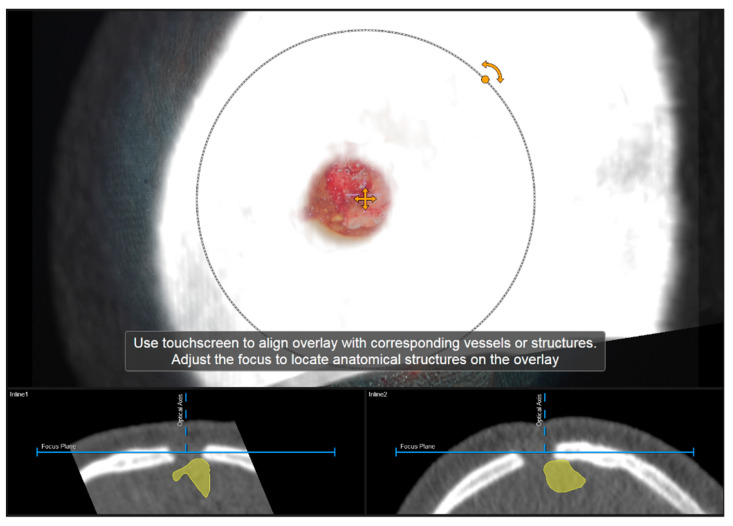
Evaluation of navigation accuracy using a CT-based MIP projection centered at a burr-hole of a prior biopsy surgery, showing a good match of image and patient data (In parallel view of MIP projection (upper part) and inline views with the recent focus plane (blue line) and the optical axis (dashed blue line) in the bottom part).
